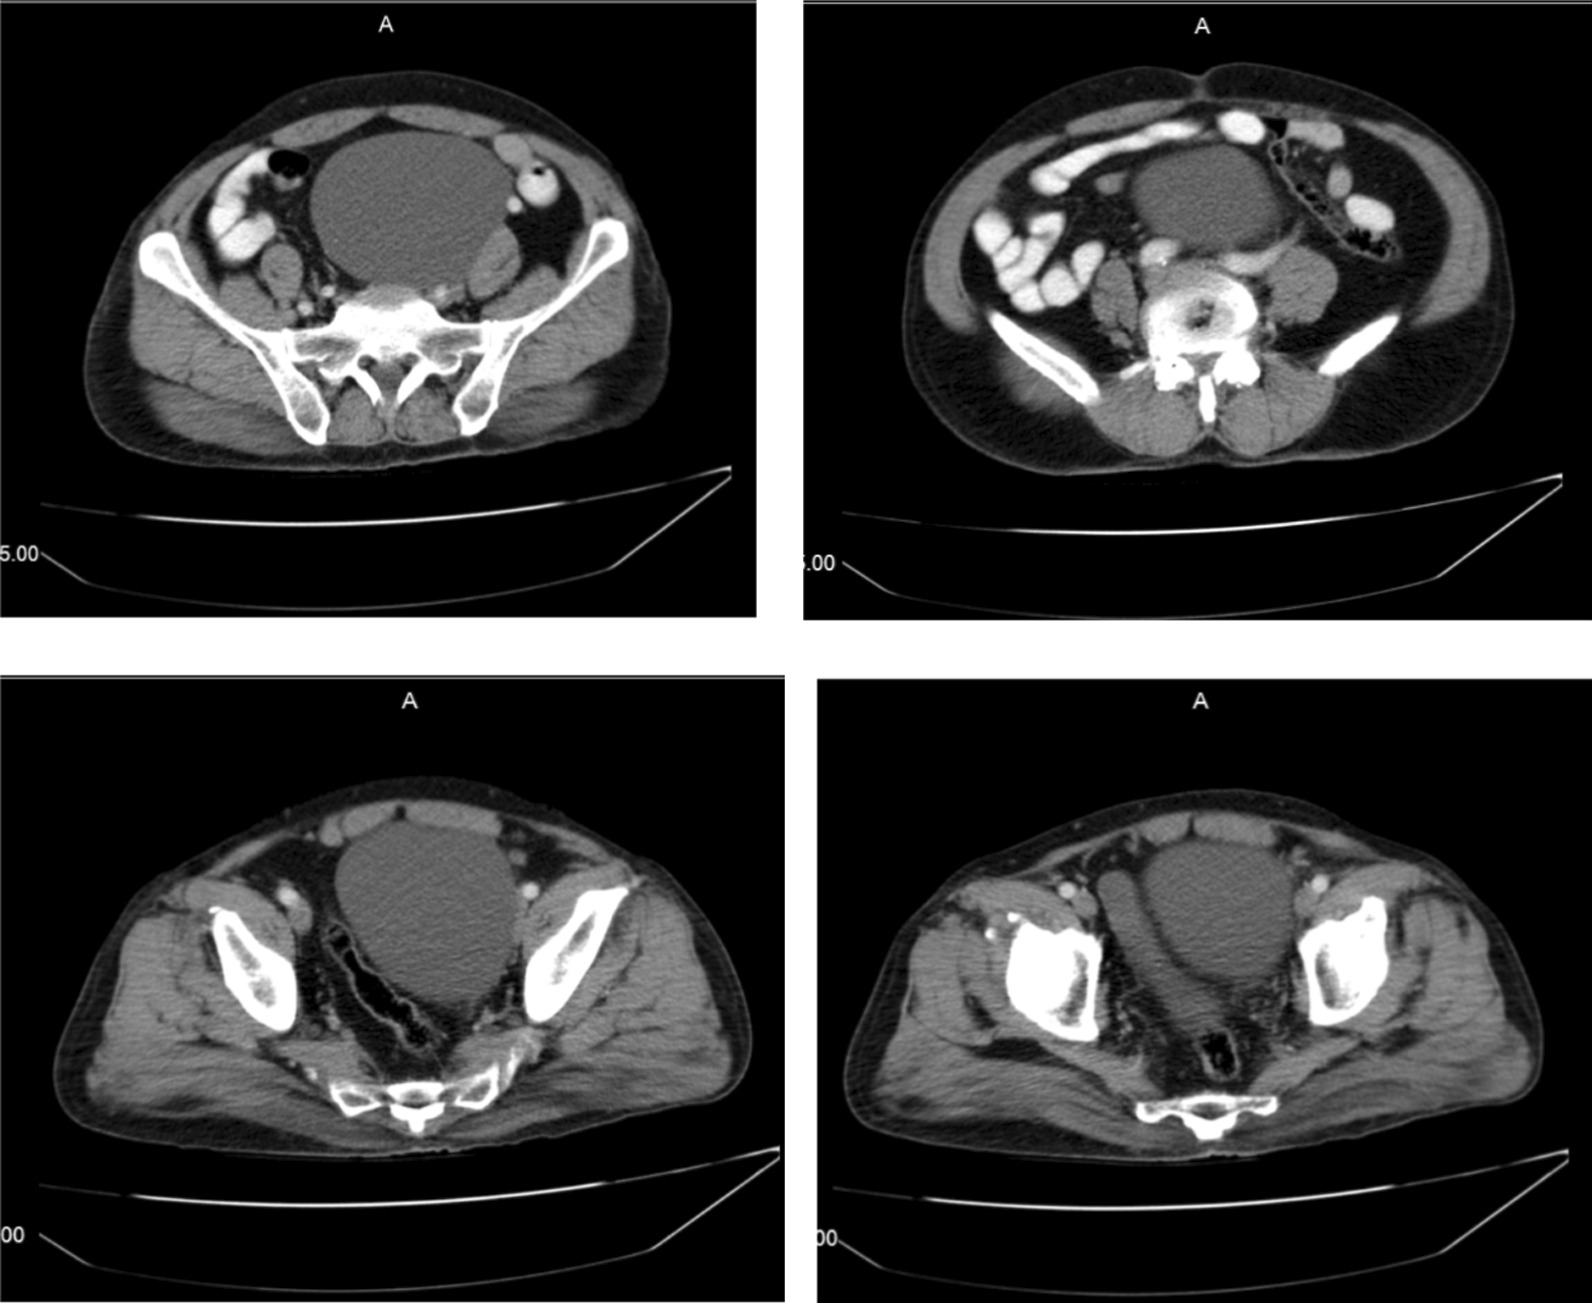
A contrast-enhanced CT scan of the abdomen and pelvic, a well-defined huge (140 × 120 mm) cystic structure within the left hemi-pelvic cavity
